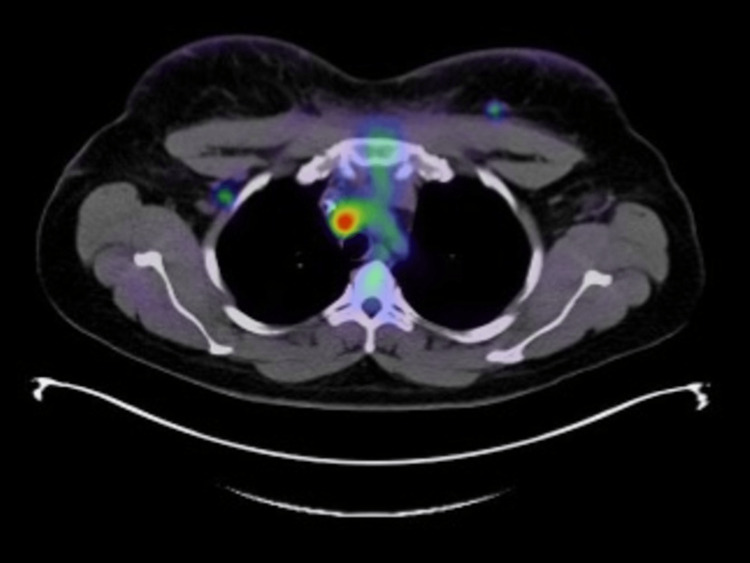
Axial PET/CT image demonstrating hypermetabolic uptake in the superior mediastinum. PET: positron emission tomography, CT: computed tomography
